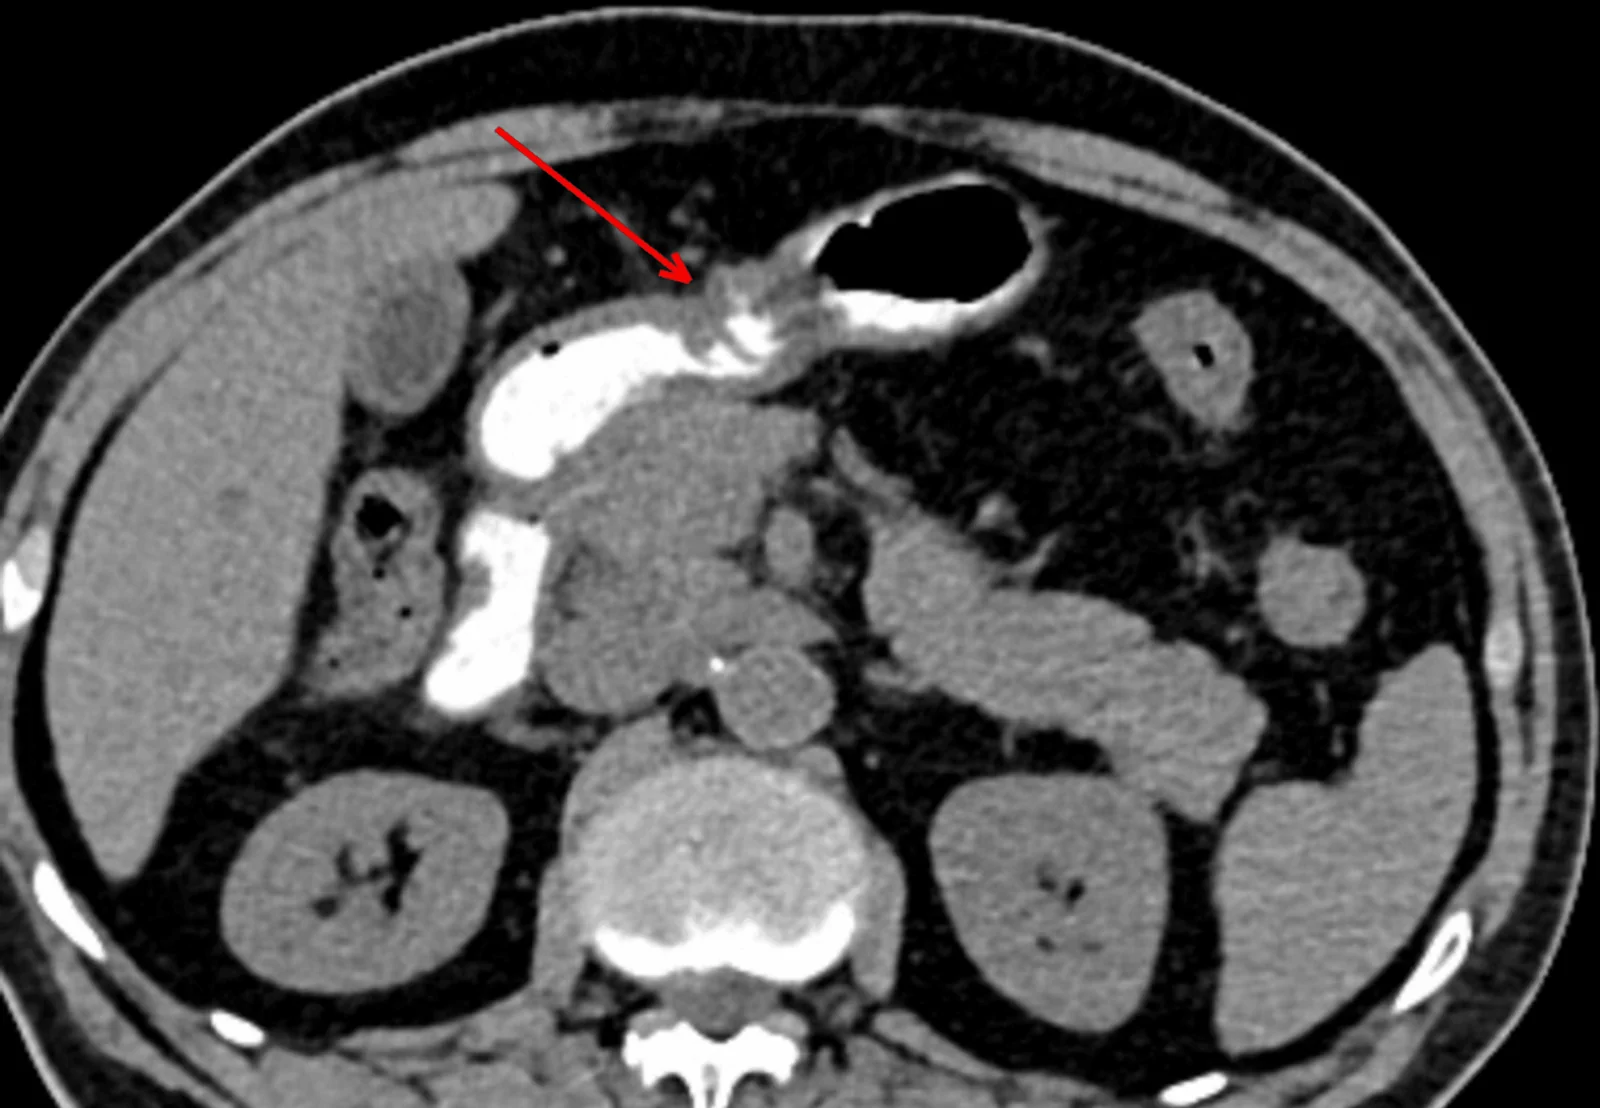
Plain-phase (non-contrast) CT of the abdomen showing a pancreatic head and uncinate process (arrow)
